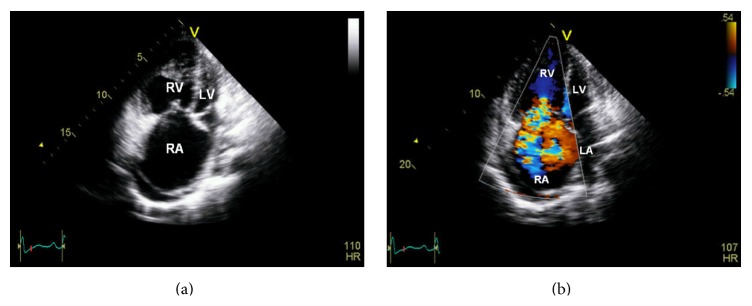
(a) Echocardiography apical view at end systole showing markedly enlarged right ventricle (RV) and right atrium (RA) and compressed left ventricle (LV). (b) Color echo Doppler image showing severe tricuspid regurgitation. LA = left atrium.
